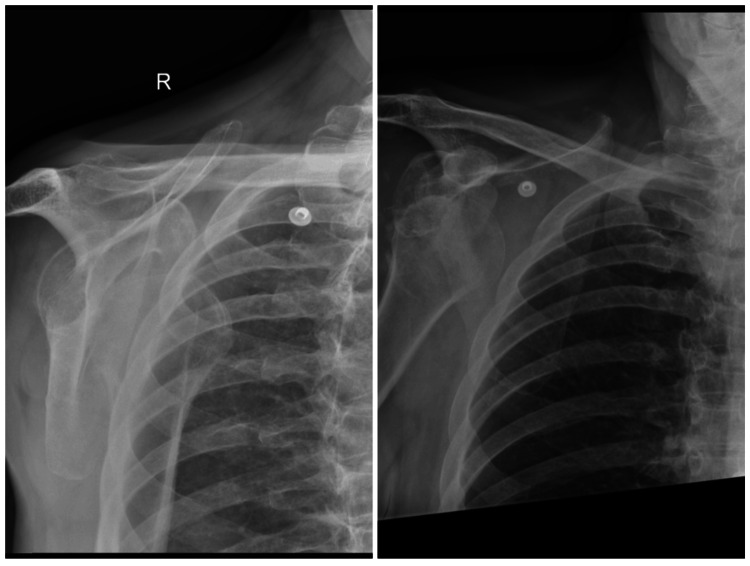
Pre-operative radiographs demonstrating chronic anterior dislocation of the right glenohumeral joint.
